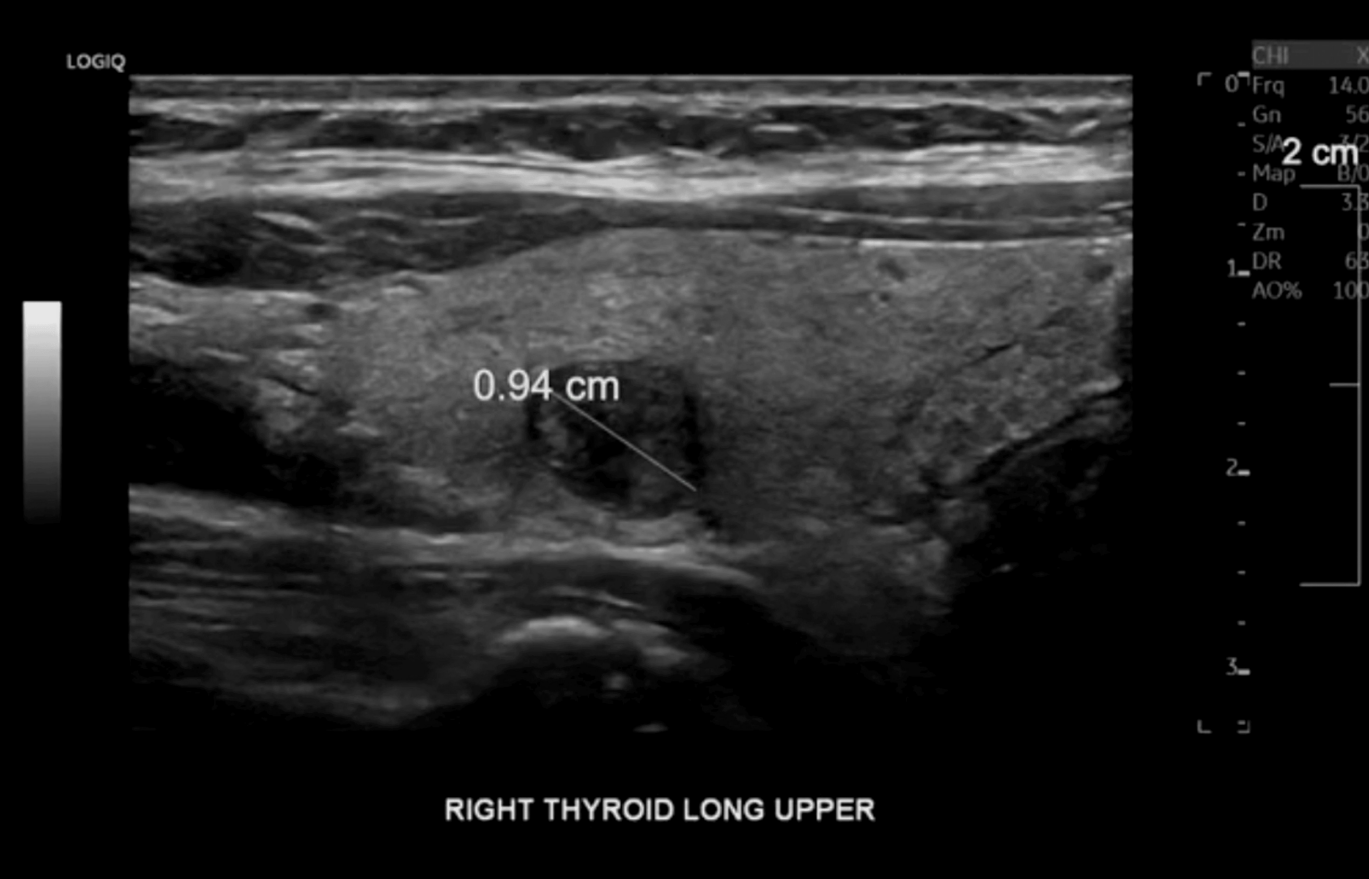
Ultrasound Imaging of Thyroid Nodule
